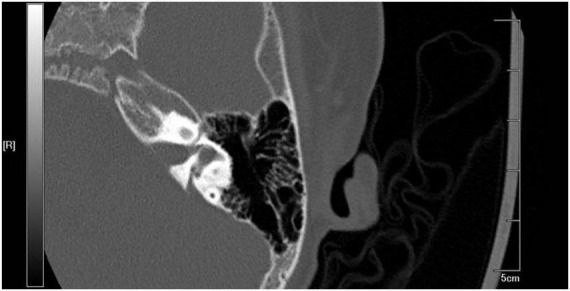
Computed tomography (CT) imaging from recruited large vestibular aqueduct syndrome (LVAS) Patient.
